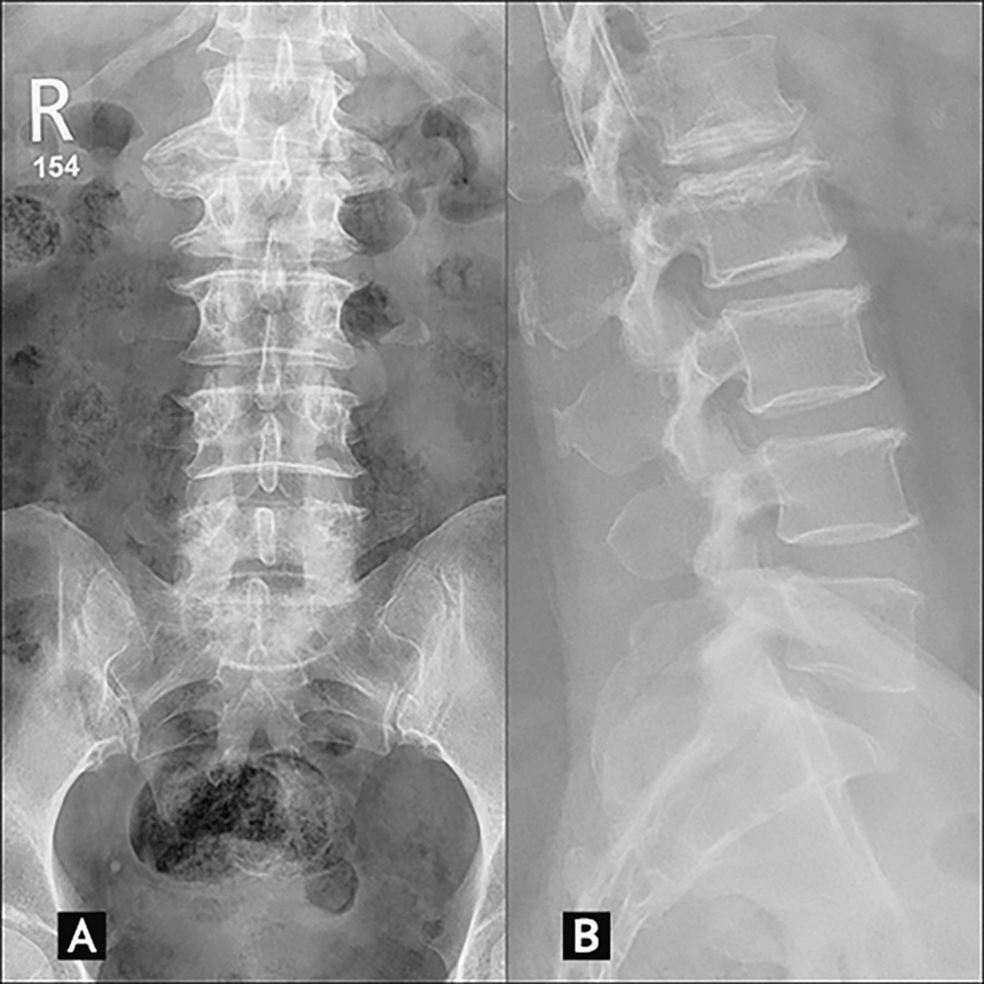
Lumbar X-ray shows at the level of L1-L2 (A) a large disc-osteophyte complex on the anteroposterior view and (B) sclerosis of endplates on the lateral view.
